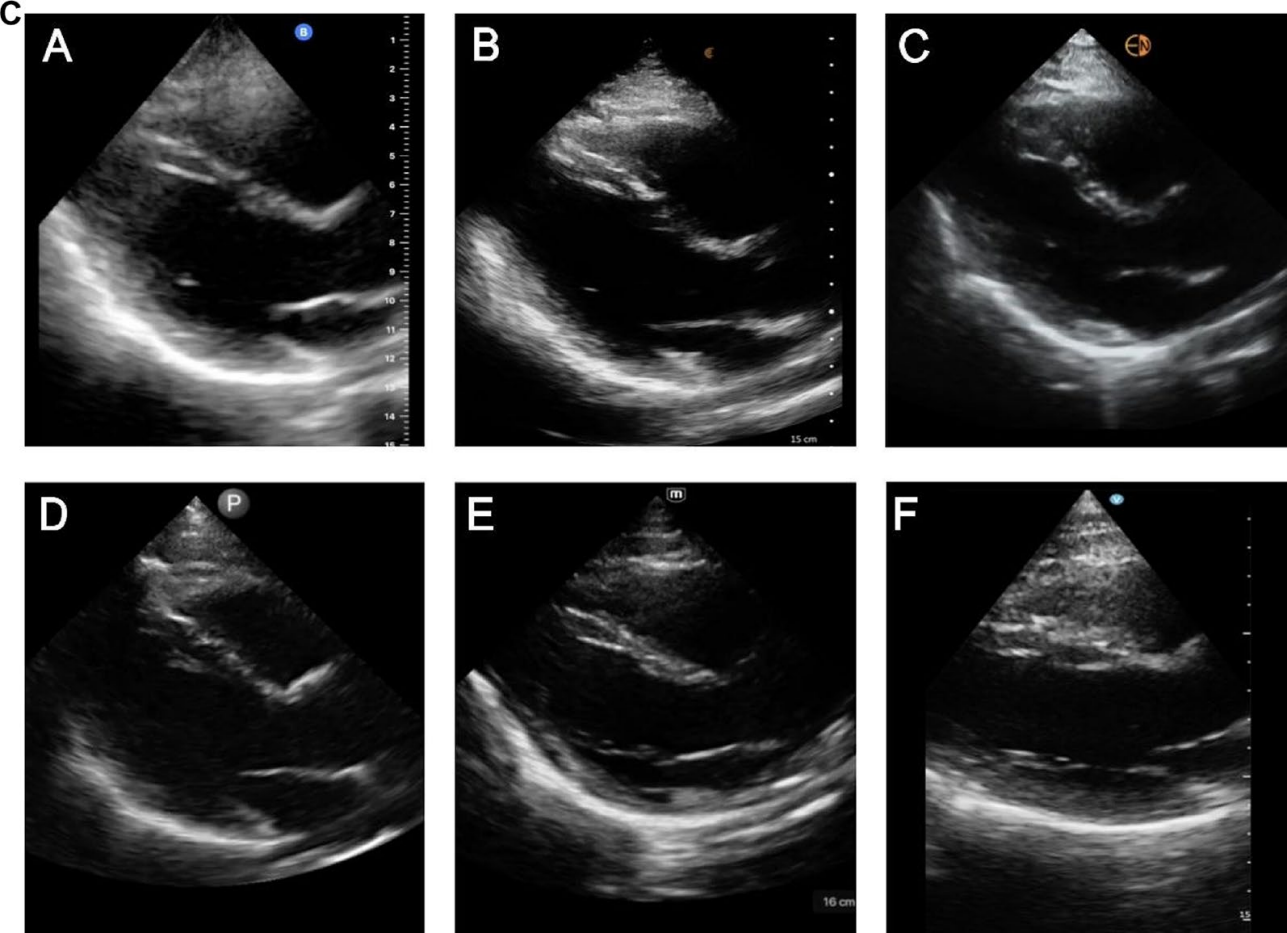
A) Cardiac Apical 4-chamber View ratings of image quality by handheld (5 domains displayed were rated on a scale from 0 to 3); B) Cardiac Apical 4-chamber View acquired from the same standardized patient in mid-diastole with the mitral and tricuspid valves open from 6 handheld devices: A Butterfly iQ +^™^, B Clarius^™^, C Kosmos^™^, D Lumify^™^, E Mindray, and F Vscan Air^™^; C Cardiac Parasternal Long-axis View acquired from the same standardized patient in early systole with the mitral valve closed and aortic valve open from 6 handheld devices: A Butterfly iQ + ^™^, B Clarius^™^, C Kosmos^™^, D Lumify^™^, E Mindray, and F Vscan Air^™^
